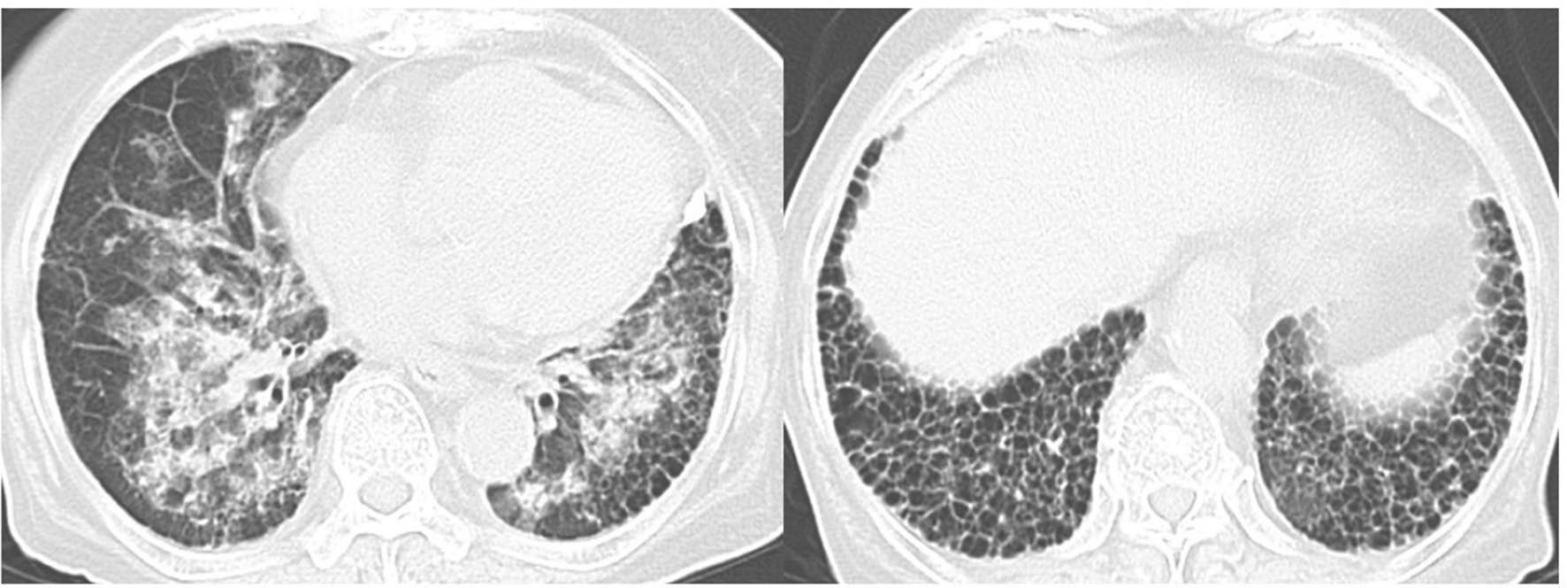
Computed tomography images of the lungs
